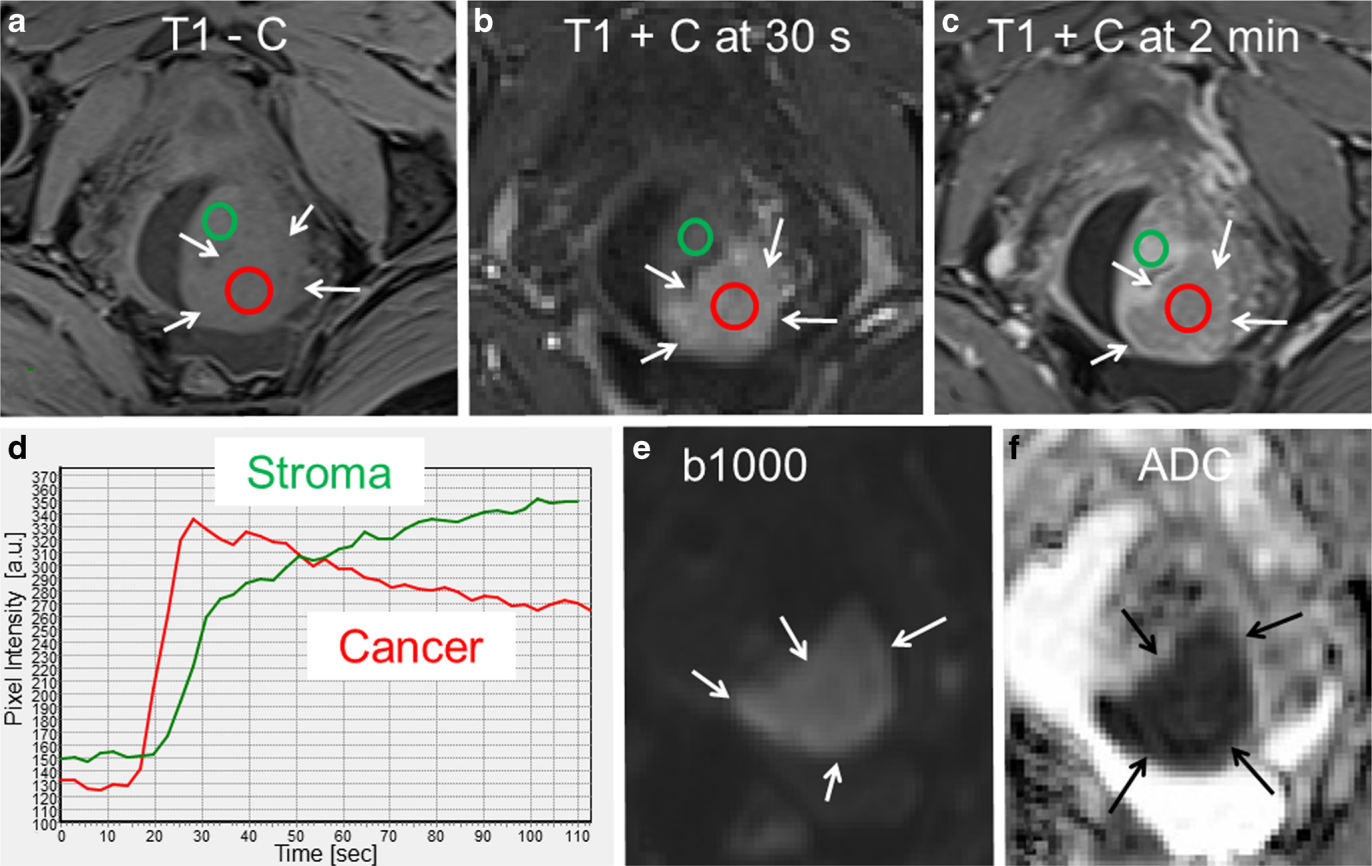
Paraaxial T1-weigthed MRI prior to contrast (a), at 30 s postcontrast (b) and 2 min postcontrast (c) and DWI (e, b = 1000 s/mm^2^) with corresponding ADC map (f) depicting a cervical lesion (arrows) in a 41-year-old woman diagnosed with squamous cell carcinoma, clinical FIGO stage 1B1 cervical cancer (same patient as in Figs. 1f, g and 3a, b). The cervical cancer tissue (arrows; red ROIs in a–c) is isointense relative to the normal stromal tissue (green ROIs in a–c) on T1-weighted MRI prior to contrast (a), hyperintense at 30 s postcontrast (b), and slightly hypointense at 2 min postcontrast (c). The corresponding time-intensity curves of cervical cancer tissue and normal stromal tissue are depicted in d. The tumor tissue also exhibits restricted diffusion with hyperintensity on high *b*-value DWI (e) and hypointensity on the corresponding ADC map (f). Due to MRI findings suggesting left parametrial invasion with disrupted stromal ring to the left (best seen in c and Fig. 1g), this patient was subjected to primary brachytherapy followed by chemotherapy (cisplatin). The patient had no signs of recurrence at 2.5 years posttreatment
